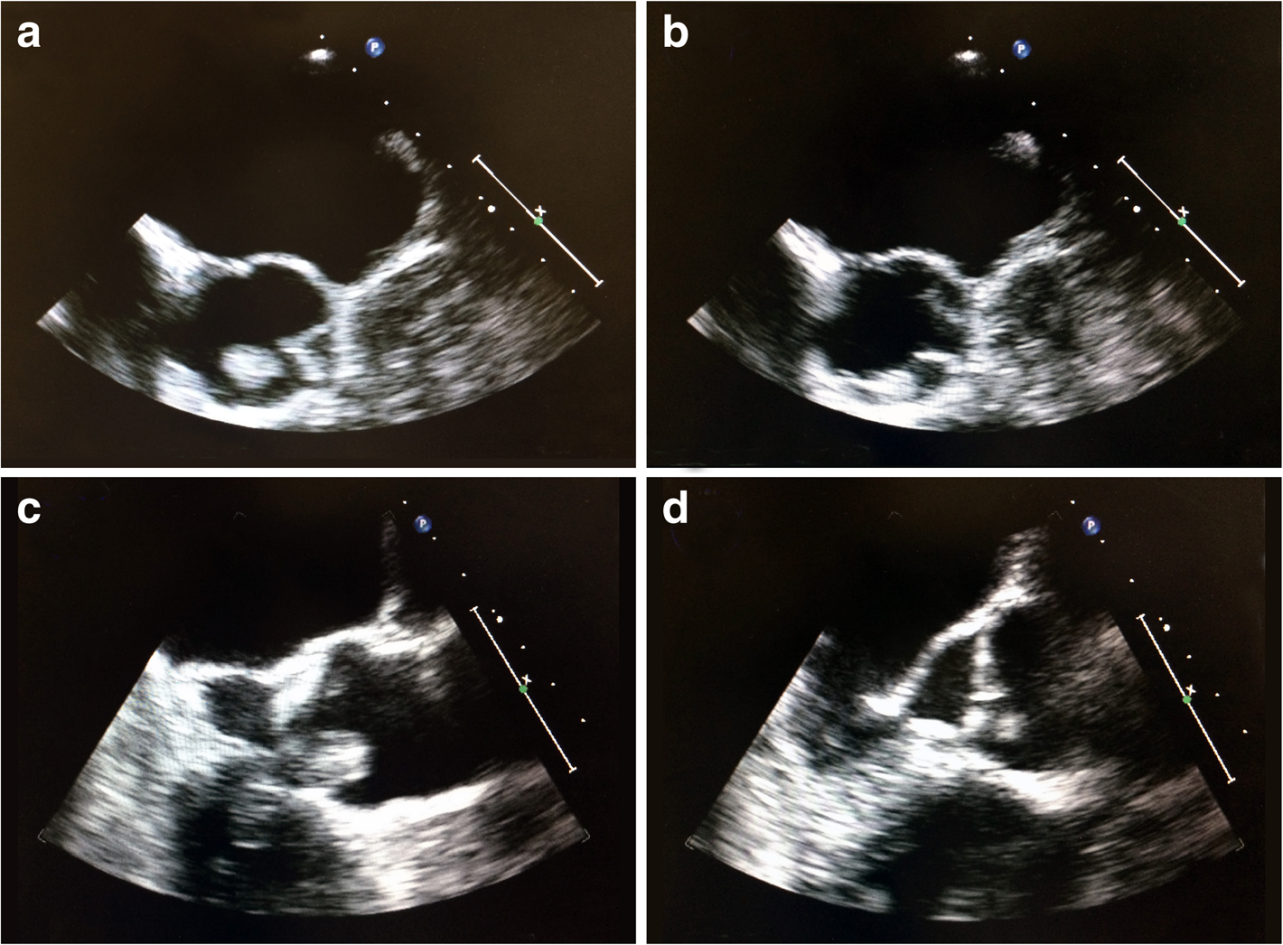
Transesophageal echocardiography. a, b Transesophageal echocardiography in the mid-esophageal view of aortic valve at long axis revealed a mobile mass 11×15 mm in diameter attached to the right leaflet of the aortic valve. It partially prolapsed in the left ventricular outflow tract of the aorta. c, d Mid-esophageal aortic valve short axis view of the papillary fibroelastoma attached to the aortic side of the right coronary cusp
